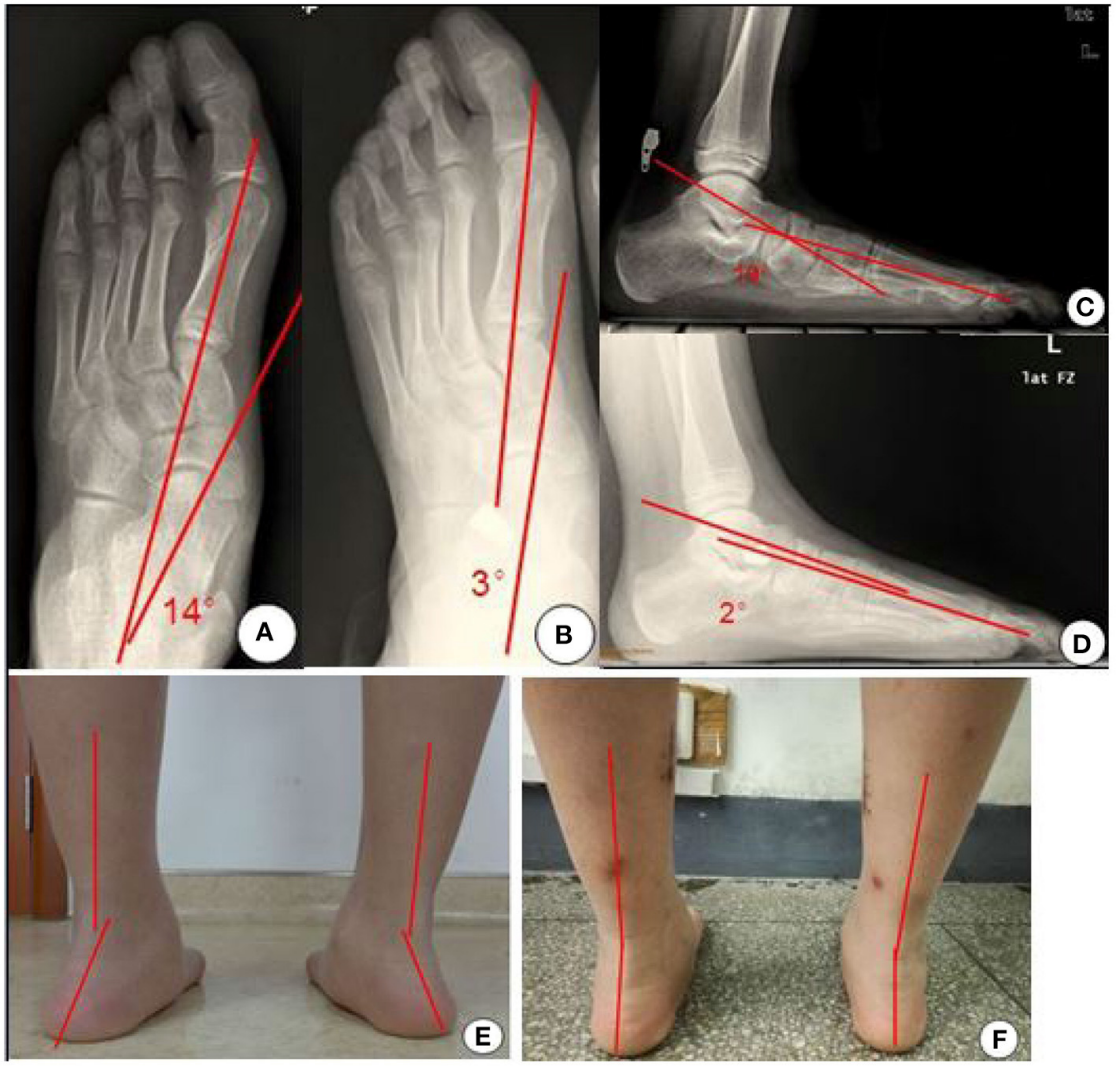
X-ray films and appearance photos before and after operation. (A) The left foot X-ray film (AP) before operation. (B) The left foot X-ray film (AP position) after operation. (C) The lateral X-ray film of the left foot before operation. (D) The lateral X-ray film of the left foot after operation. (E) The posterior foot line of force before operation. (F) The posterior foot line of force after operation.
